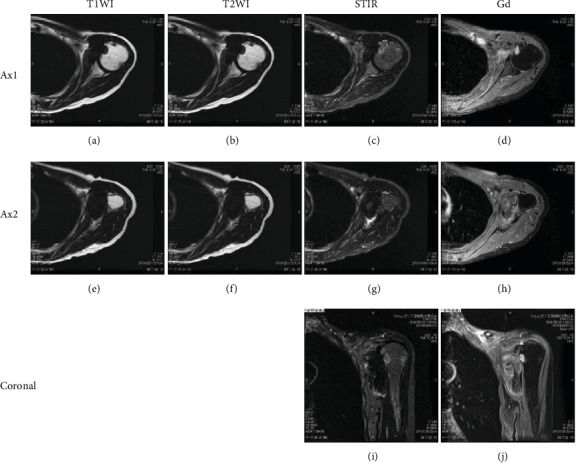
MRI images of the DFB in the left axilla with humeral invasion. (a–d) Raw axial images of the lesion with invasion of the underlying humerus. (e–h) Raw axial images of the lesion at its maximum diameter. (i–j) Raw coronal images of the lesion. First column (a, e), T1-weighted images. Second column (b, f), T2-weighted images. Third column (c, g, i), T2-weighted fat-suppression images. Fourth column (d, h, j), postcontrast T1-weighted images with fat suppression. The abbreviations: T1WI: T1-weighted images; T2WI: T2-weighted images; STIR: short TI inversion recovery; Gd: gadolinium-enhanced fat-suppressed T1-weighted images; Ax: axial images.
